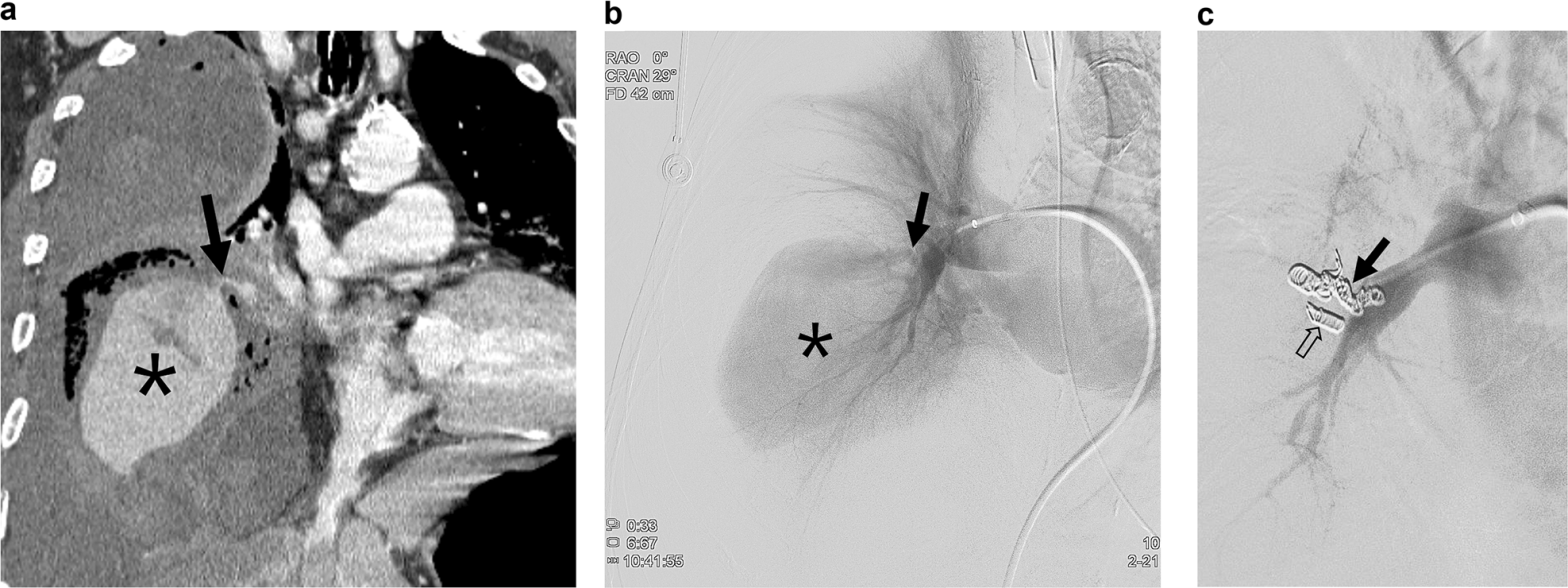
A 78-year-old male was admitted with fever and chills 2 months after radiation therapy for small cell lung cancer in the right lower lobe. a On the chest CT, a huge pulmonary artery pseudoaneurysm (PAP) (*) surrounded by necrotic lung tissue, suggestive of necrotizing pneumonia, was identified in the right middle lobe of the lung. The leaking point of the pseudoaneurysm from the pulmonary artery (arrow) can be noticed. b Similar to the CT findings, a huge PAP (*) and a short neck of PAP (arrow) can be visualized on the pulmonary arteriography. c Embolization of the pseudoaneurysm neck was performed with multiple microcoils (arrow). Complete exclusion of the PAP can be observed, with the filling of PAP no longer visible on the pulmonary arteriography. Some coils appear to have migrated into the PAP (open arrow) or protruded into the pulmonary artery owing to the short pseudoaneurysm neck. However, the patient died from sepsis after 3 weeks
